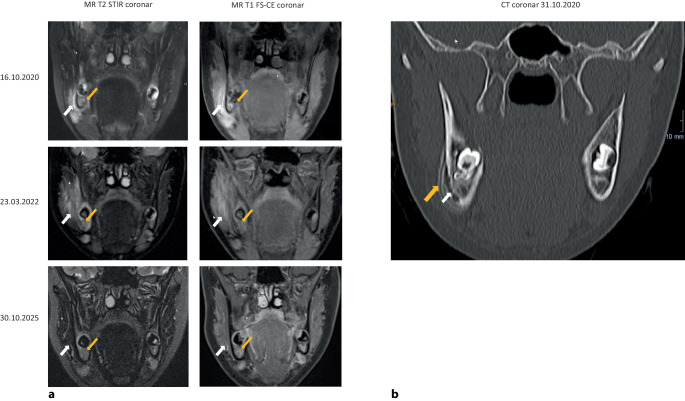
a*Obere Zeile* Die MR-T2-Sequenz koronar mit STIR-Fettunterdrückung (MR T2 STIR cor; *linke Spalte*) vom 16.10.2020 zeigt ein Knochenödem (*Pfeil*, *gelb*) und ein unmittelbar angrenzendes Weichteilödem (*Pfeil*, *weiß*). In der MR-T1-Sequenz mit Fettsättigung koronar nach Kontrastmittel (MR T1 FS-CE cor; *rechte Spalte*) kommen das Knochenödem und das Weichteilödem kontrastreicher und ausgedehnter zur Darstellung (entsprechende *Pfeile*, *gelb* bzw. *weiß*). *Mittlere Zeile* Die MRT vom 23.03.2022: Die MR-T2-STIR cor zeigt im Vergleich zur Voruntersuchung weiterhin ein deutliches Knochenödem sowie eine flächige Zunahme der Weichteilödemausdehnung. Die MR-T1 FS-CE cor zeigt hier zusätzlich ein stärkeres KM-Enhancement der knöchernen Strukturen und der umgebenden Weichteile. (*Pfeilmarkierungen* analog obere Zeile). *Untere Zeile* MRT vom 30.10.2025: MR T2 STIR cor (*linke Spalte*) und MR T1 FS-CE cor (*rechte Spalte*): Die knöchernen Strukturen, das Periost und umgebende Weichgewebe zeigen deutlich rückläufige Befunde. Hier finden sich weder in der STIR-Sequenz noch in der KM-Serie signifikant pathologische Signale. (*Pfeilmarkierungen*, analog obere Zeile). b CT vom 30.10.2020: Die CT zeigt eine Arrosion der Kompakta (*weißer Pfeil*) sowie eine lamelläre Periostreaktion dort angrenzend (*gelber Pfeil*)
